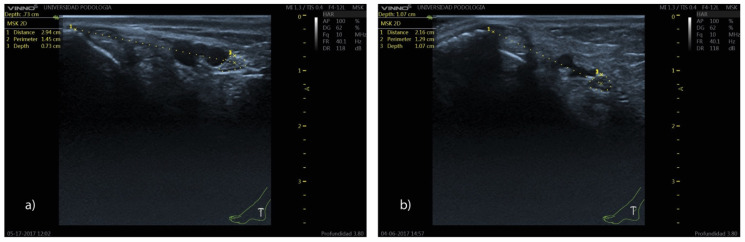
Ultrasound image showing the position of the tibial nerve at the retromalleolar level in men (a) and women (b). Note that the distance from the bony reference point to the center of the tibial nerve is greater in men (a) (2.94 cm vs. 2.16 cm), whereas the depth relative to the skin surface is greater in women (b) (0.73 vs. 1.07 cm).
